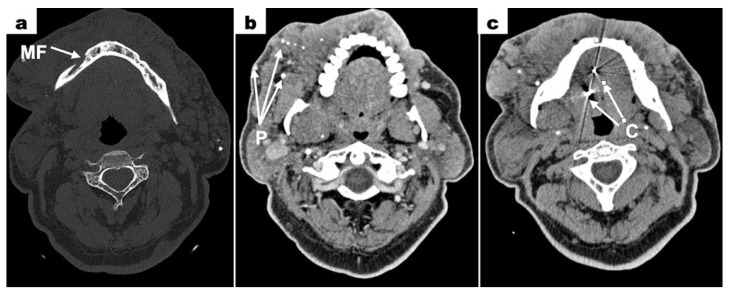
Contrast-enhanced CT scans; (a) lack of a defined boundary between the mental foramen and the vascular malformation mass (MF = mental foramen); (b) extensive areas of densification with calcifications in the blood vessels of the vascular malformation mass (P = phleboliths); (c) metallic foreign bodies in the projection of the tongue—clips used in a treatment to tether the tongue in childhood (C = clips).
